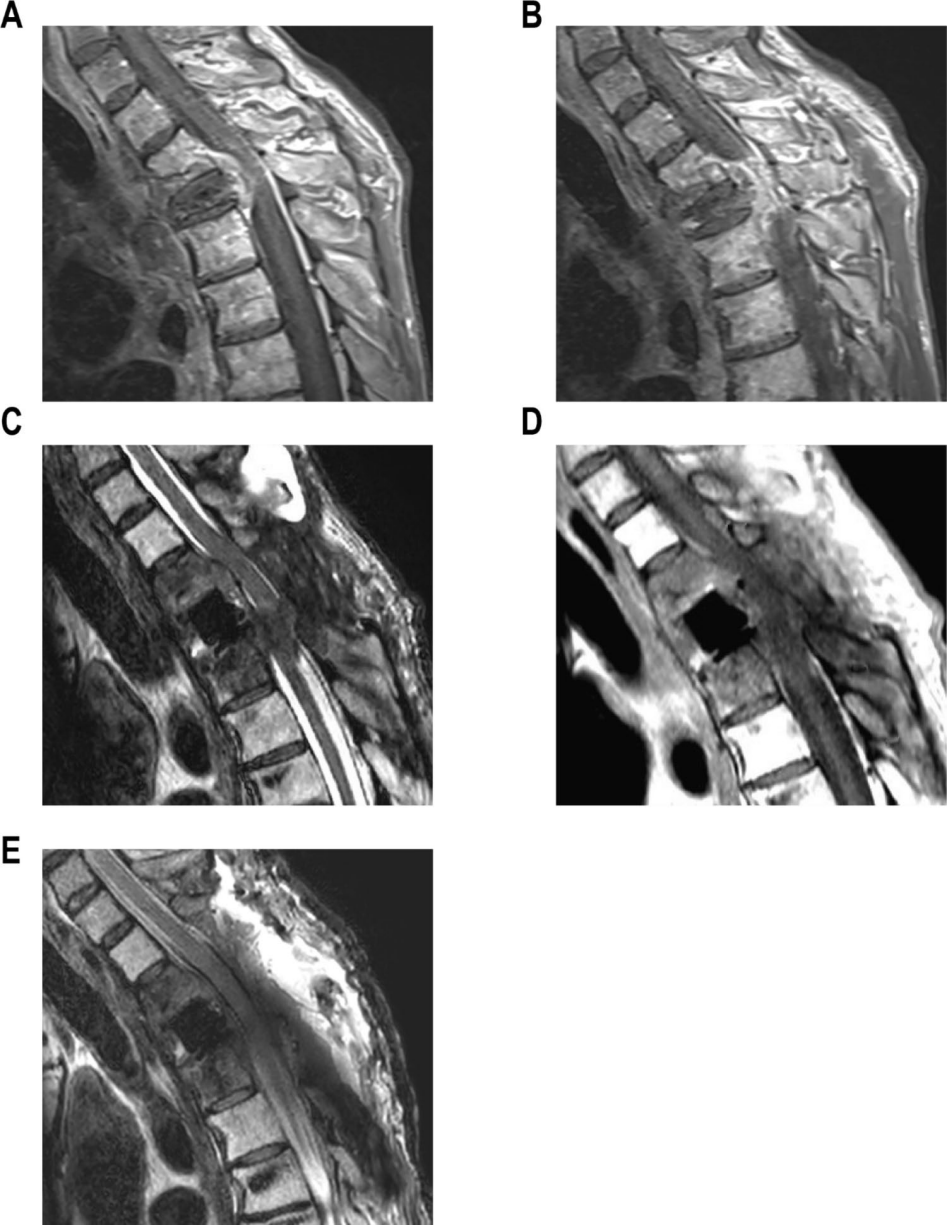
Case 3: Pre- (A, B) and postoperative (C, D, E) sagittal MRI T2 weighting (A, C, E) and T1 with KM weighting (B, D)
